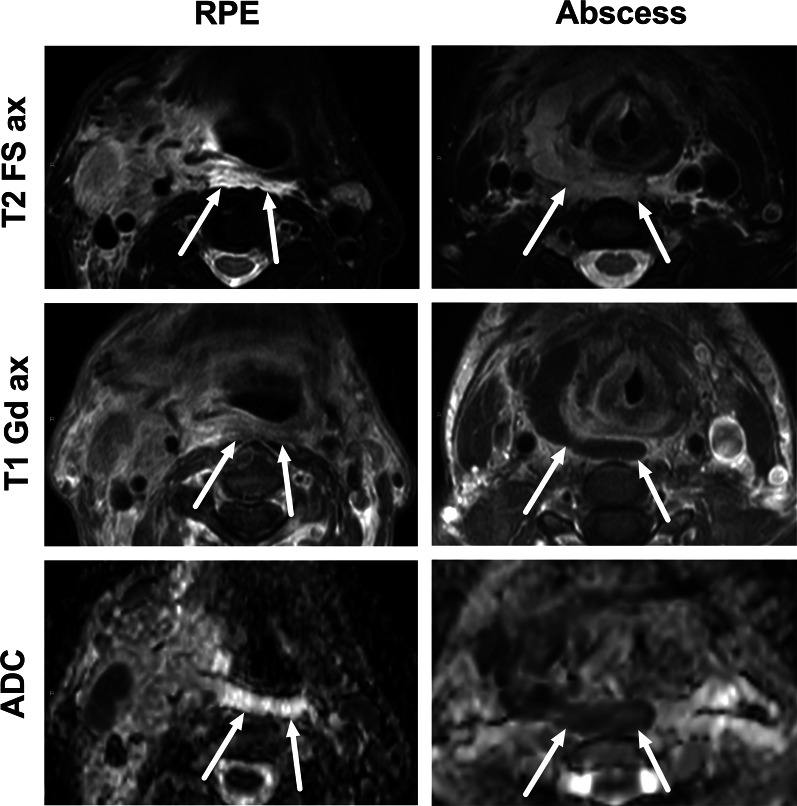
Differentiating between RPE and a retropharyngeal abscess: RPE is usually very bright on T2-weighted images, whereas abscess has an intermediate signal. Enhancement of RPE is variable, whereas an abscess does not enhance. Finally, the key is ADC: bright in RPE, dark in abscess. Arrows denote collections. Image modified from ref. [12]
